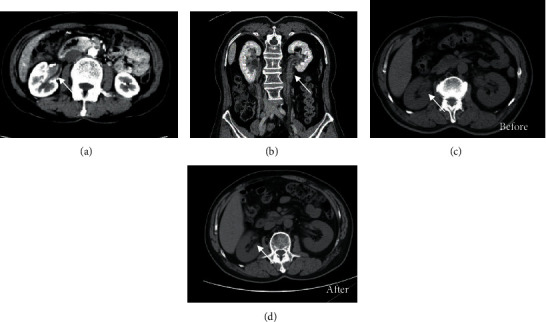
(a) CT scan revealed renal pelvis mass and thickening wall without enhancement (arrow). (b) Ureter mass without enhancement of IgG4-related ureter disease (arrow). (c) Repeated CT scan showed remission of thickened right renal pelvis wall after 3-month treatment. Arrow pointed at the pelvis lesion.
